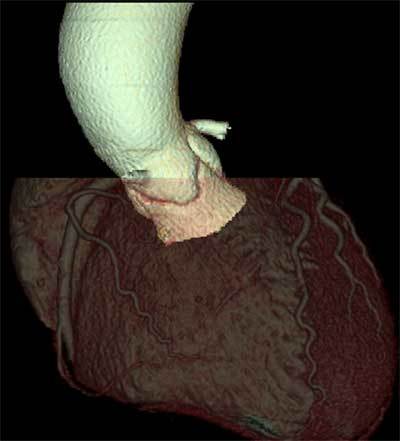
Volume-rendered CT image of the heart, indicating the double-oblique orientation of the aortic root and valve. As such, it is clear that a true 3D imaging modality like CT can provide additional benefits over CT in correctly assessing the aortic root anatomy and size in the pre-procedural TAVI work-up.
